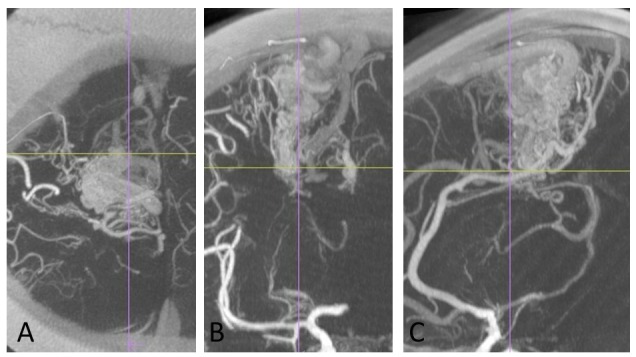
Three-dimensional evaluation of an arteriovenous malformation. The 3-D fine structures of an arteriovenous malformation in the right frontal lobe reconstructed from axial images of rotation angiography with the DICOM image analysis. The feeding arteries, nidus, and draining veins are clearly shown, as the intranidal structures. A: axial, B: coronal, C: sagittal view. The original 1 mm-axial image is used for true image fusion of angiography to thin-sliced CT for radiosurgery.
